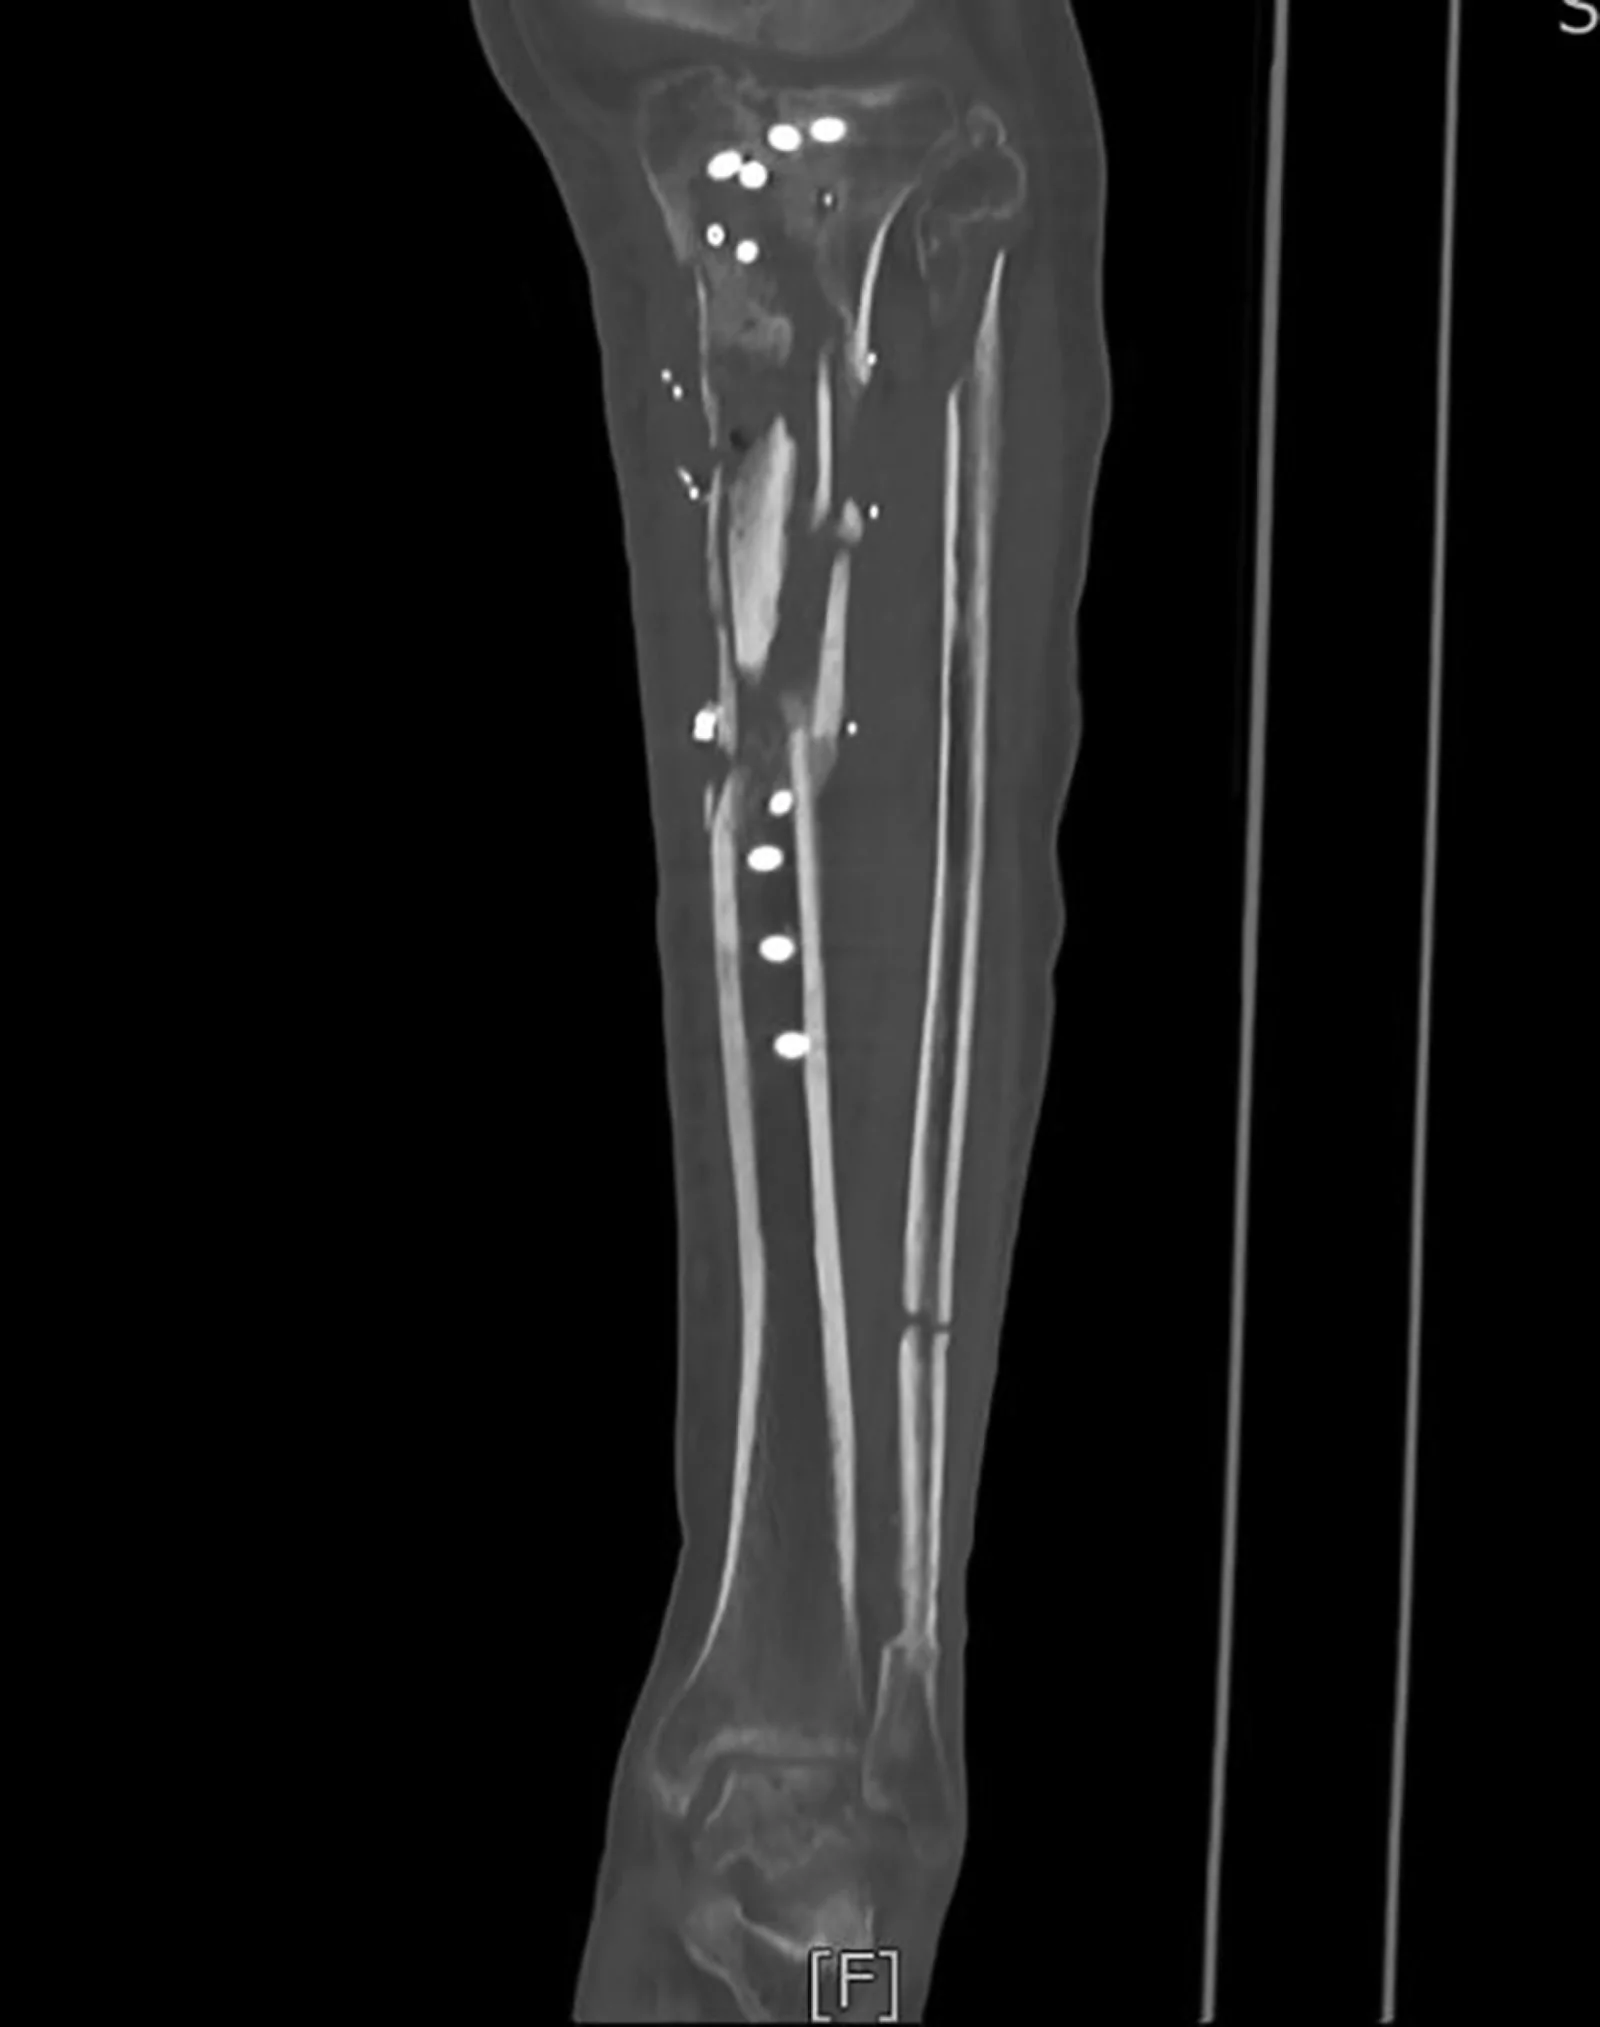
Preoperative imaging upon admission. A computed tomography (CT) scan of the left tibia shows nonunion at the fracture site with surrounding bone atrophy and sclerosis, along with retained internal fixation implants. These findings are consistent with a diagnosis of chronic post-traumatic osteomyelitis.
